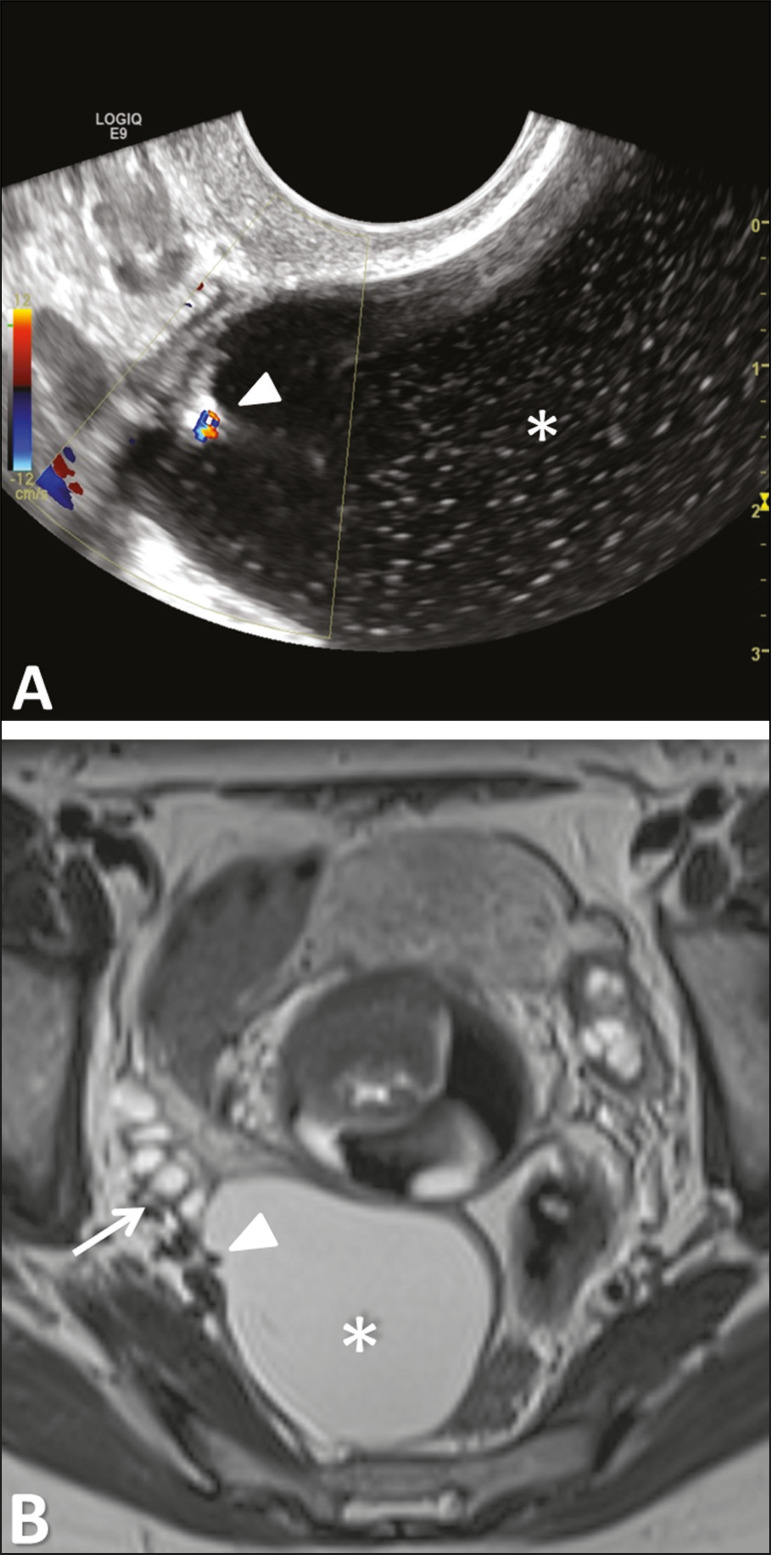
Serous borderline tumor of the left mesosalpinx in a 35-year-old woman. A: Transvaginal ultrasound showing a cystic tumor (asterisk) with a papillary projection (triangle) found to have vascularization with color Doppler. B: Axial T2-weighted imaging showing the cystic tumor with a single papillary projection, demarked from the right ovary (arrow).
